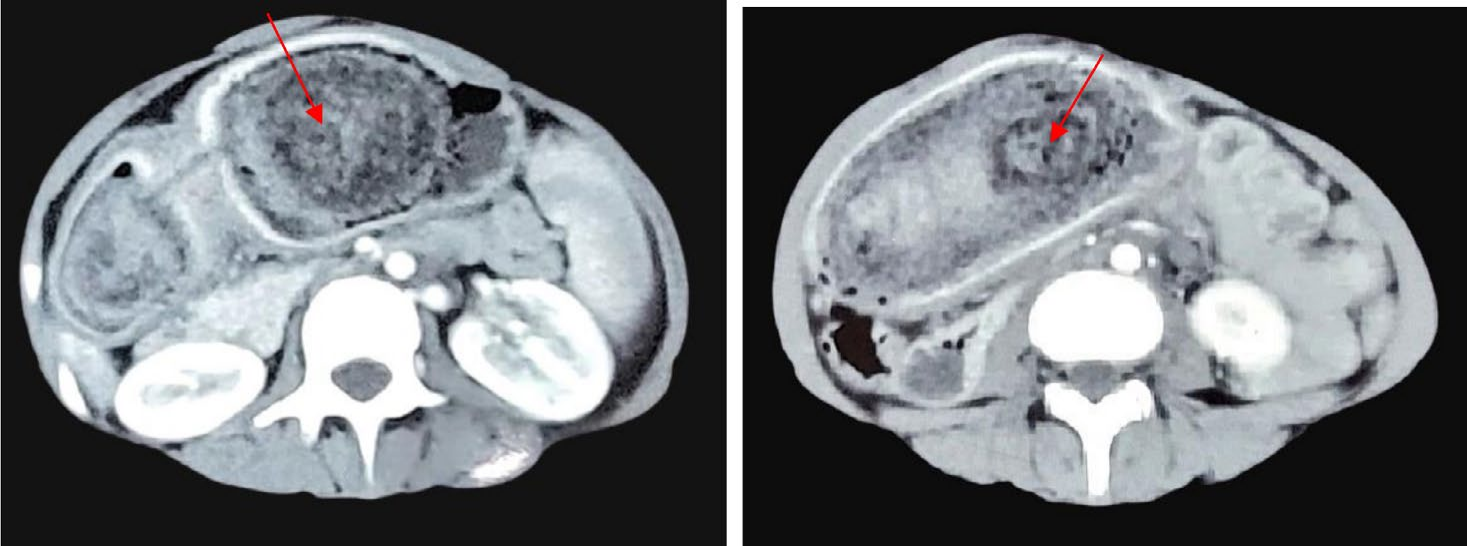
Contrast enhanced CT imaging of a gastric trichobezoar: Axial views demonstrating intraluminal mass with mottled gas pattern (shown by red arrows).
